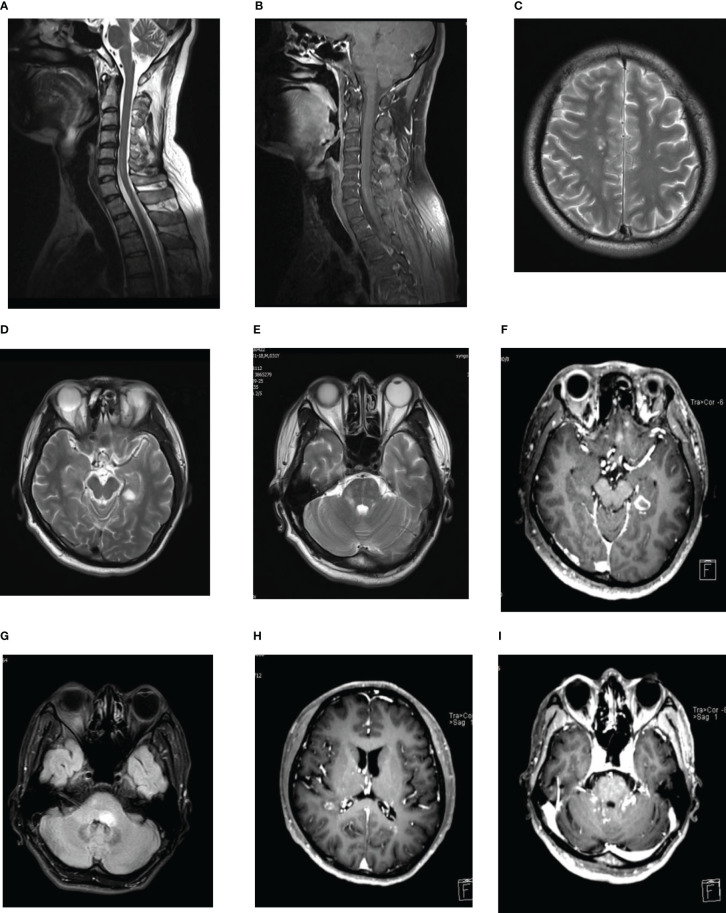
(A, B) Spinal cord MRI showed T2-hypertense lesions at C5-6 (A) with slight Gd enhancement (B). (C–F) Multiple lesions on MRI of the brain. T2-hypertense in right center semicovale, lateral paraventricular, pons, left temporal lobe, with an obvious contrast-enhancing lesions in the left temporal lobe. (G) Brain MRI revealed a new T2-hyperintense lesion located in left pons with no-contrast enhancement. (H, I) Gd-enhanced MRI showed punctate and curvilinear enhancing lesions involving the right semi-oval center areas, the anterior and posterior angles of lateral ventricle, pons and bilateral cerebellar hemisphere in the right hemispheres of hemispheres, the anterior and posterior angles of lateral ventricles, pons and left cerebellar hemispheres, without evidence of leptomeningeal enhancement.
